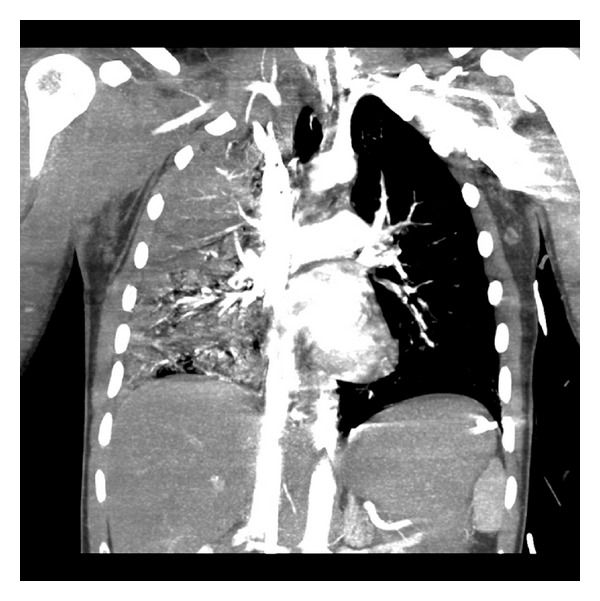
Computed tomography (CT) of the chest demonstrates extent of acute pulmonary injury.
